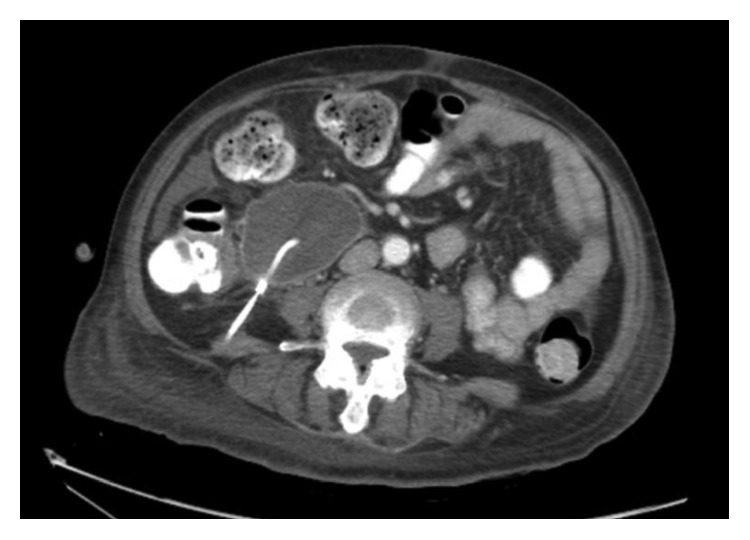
CT scan of the abdomen showing one of the pancreatic abscesses with drain placed.
